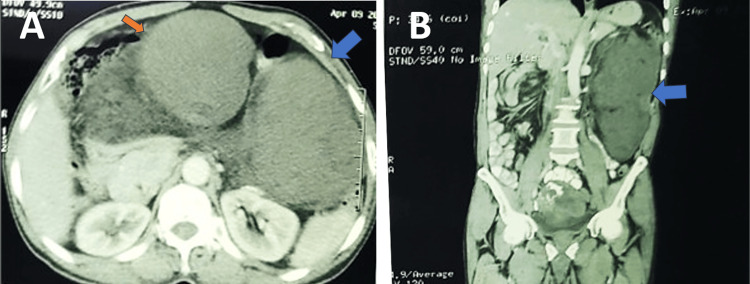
(A) Axial section of CT abdomen showing the tumor with the surrounding structures. Orange and blue arrows indicate lobules of the lesion. (B) Coronal section of CT abdomen showing the tumor (blue arrow) and its relation with the surrounding structures.
